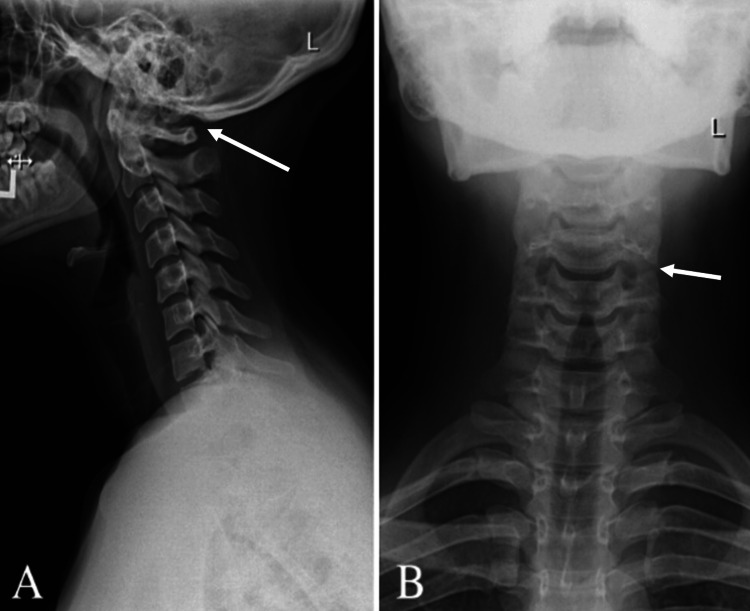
Radiograph of the cervical spine (A) lateral view and (B) anterior-posterior view demonstrating maintained vertebral body heights, disc spaces, and facet joints with mild reversal of the usual cervical lordosis without spondylolisthesis and maintained atlantodental articulation.
